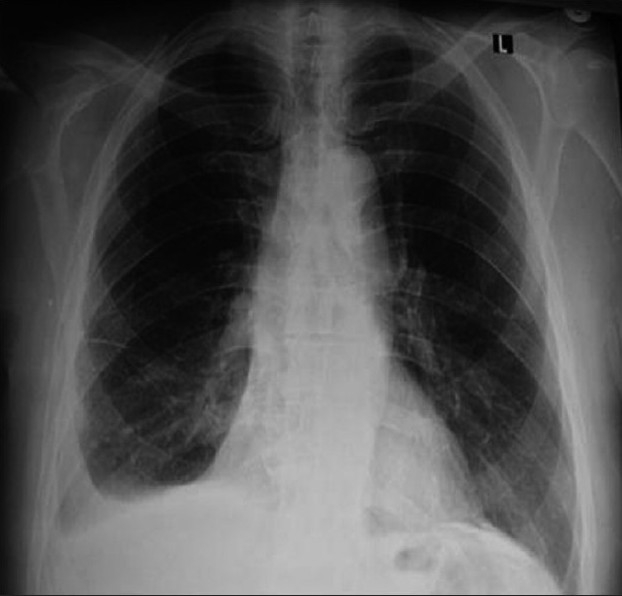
Posteroanterior chest X-ray shows minimal right-sided pleural effusion; there is left-sided pleural calcification
